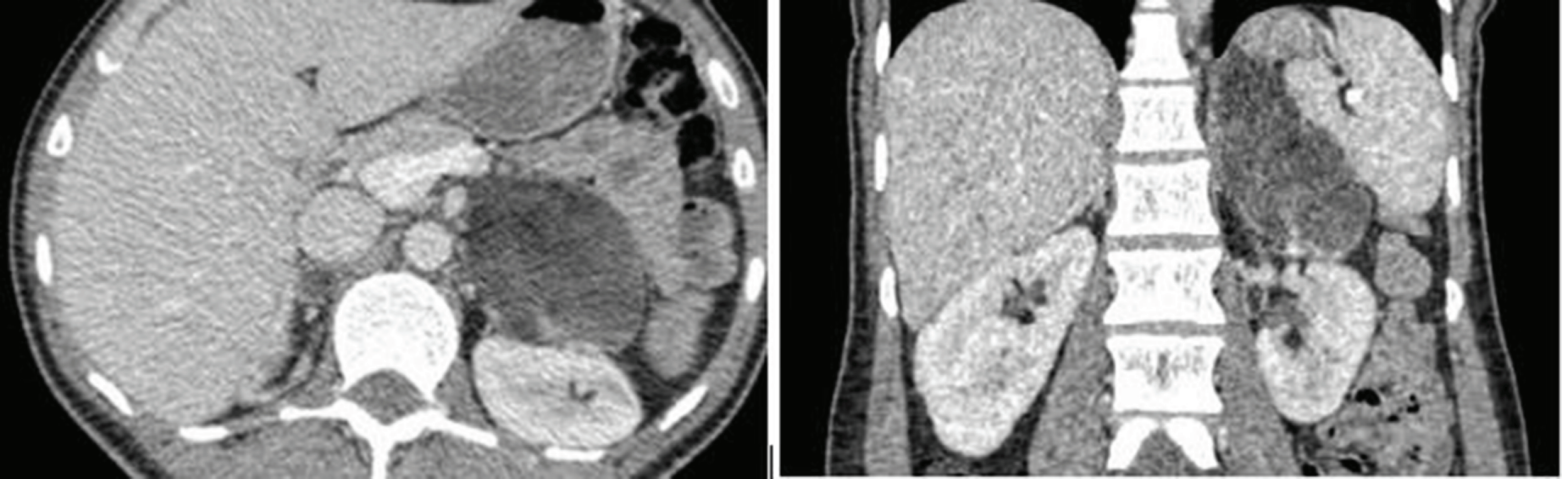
Computed tomography images of the left adrenal mass (74 x 55 mm in size) in axial (A) and coronal (B) views.
